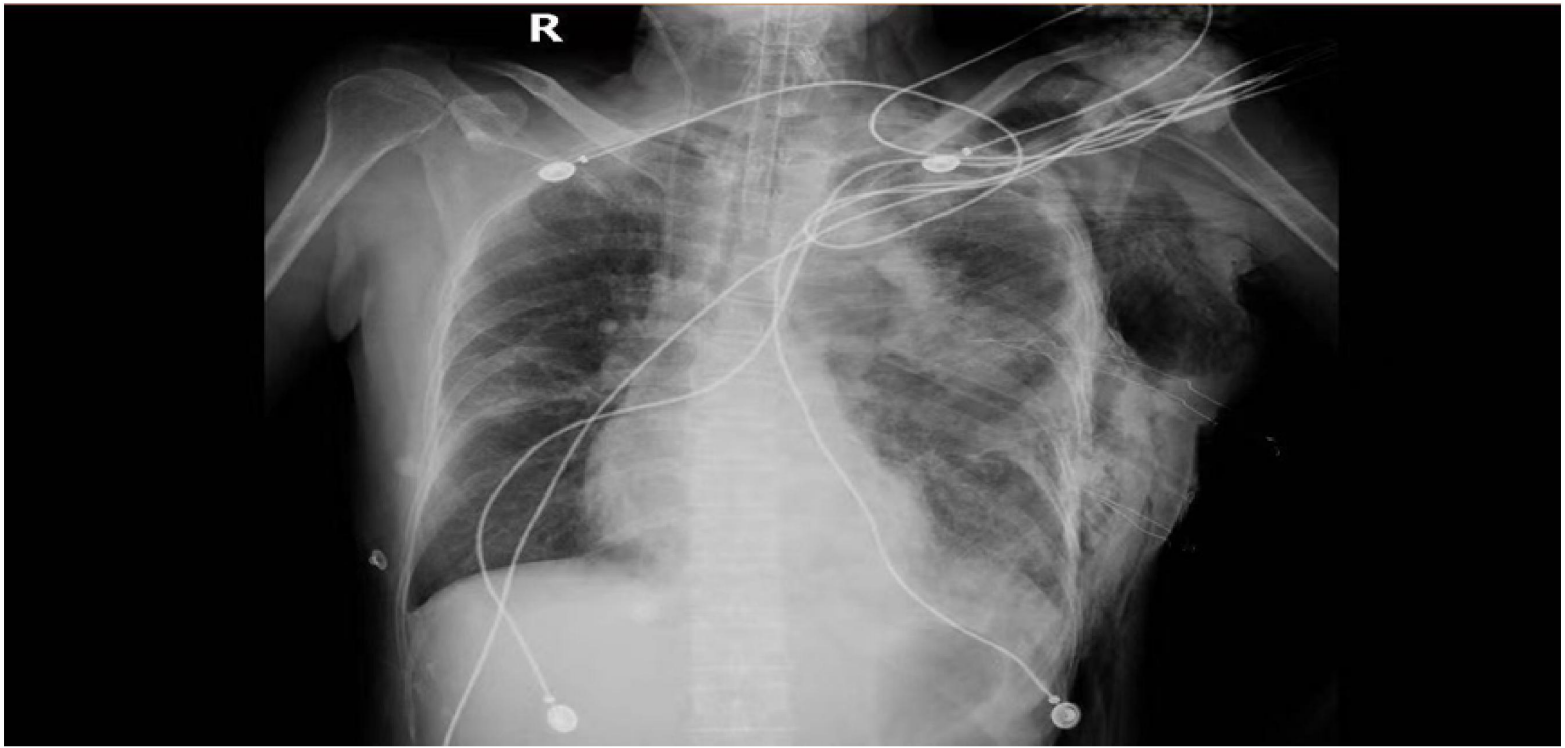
Chest X-ray of the patient 2 hours after the operation. (2023.3.29).
